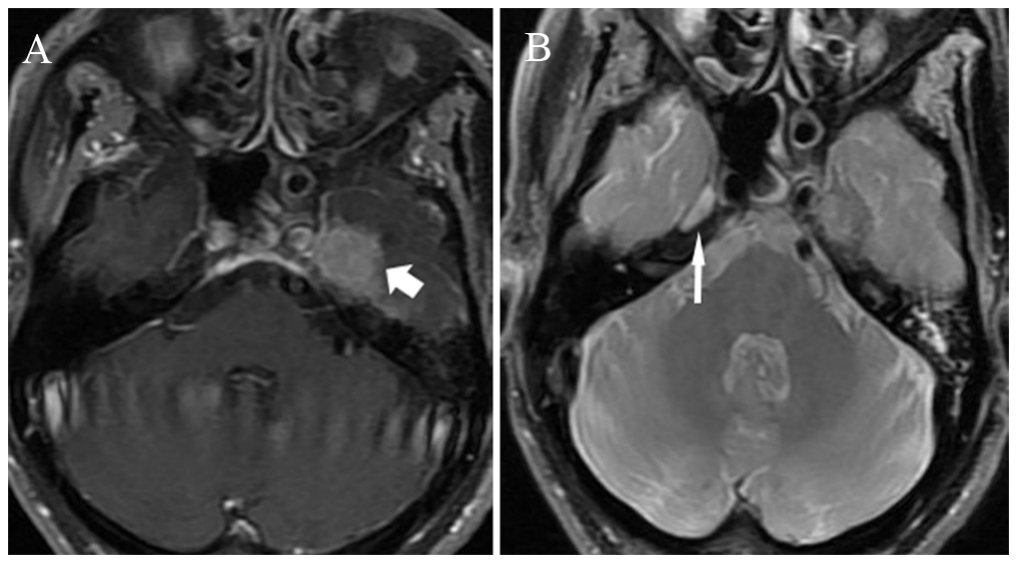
72-year-old man with nasopharyngeal carcinoma with cranial nerve invasion: (A) enhanced axial T1-weighted image with fat suppression shows the effacement of the left gasserian ganglion (short arrow); (B) axial PD weighted imaging shows the normal right gasserian ganglion (long arrow).
